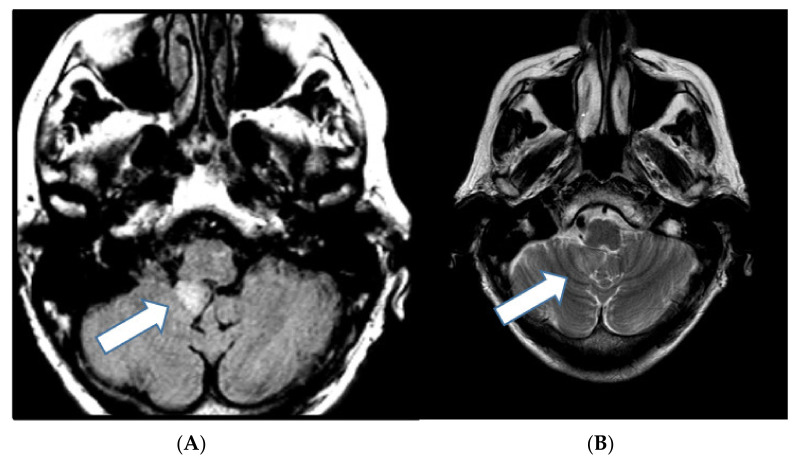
A 66-year-old woman with a history of clinical Cowden syndrome and pathologic *PTEN*-mutation-associated meningioma presenting with headache. (A) Axial FLAIR magnetic resonance image demonstrates a hyperintense oval mass measuring 13 mm at the inferior right cerebellum (arrow), encroaching on the fourth ventricle. (B) T2-weighted images demonstrate classic “tigroid” appearance representing dysplastic gangliocytoma (Lhermitte–Duclos disease).
